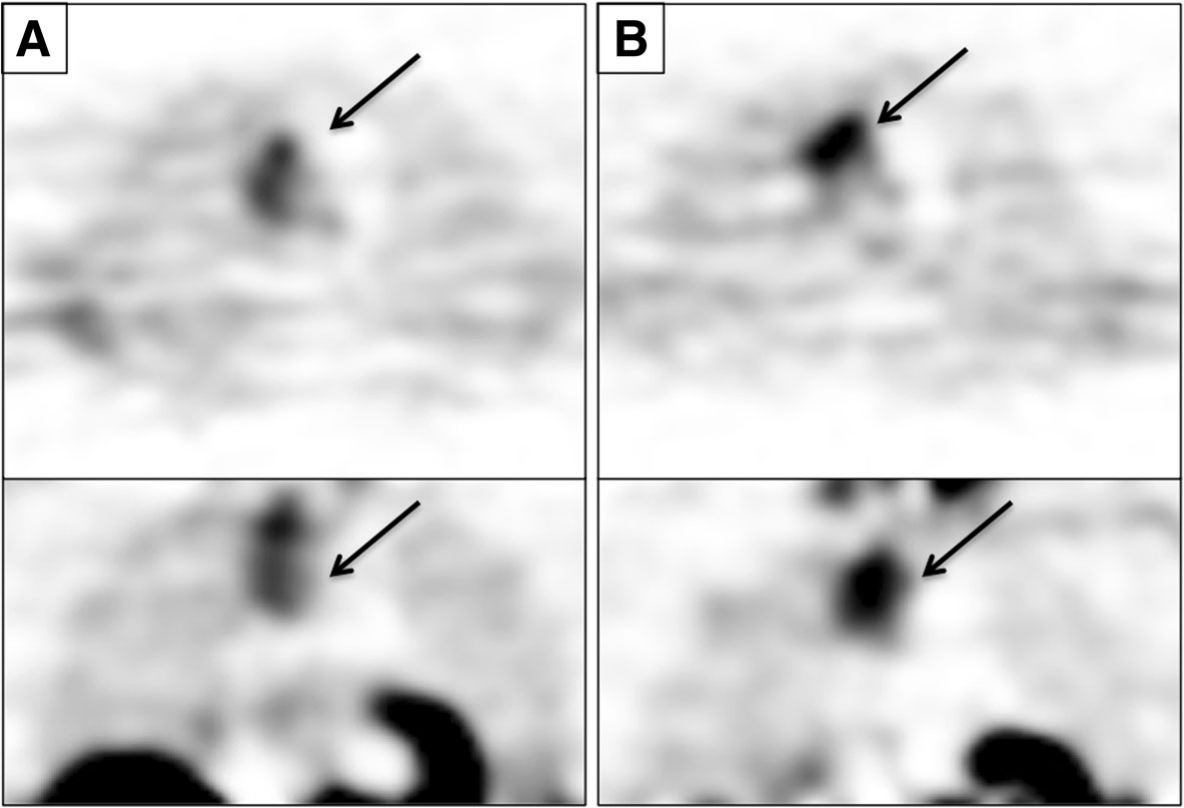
Tumor ^18^F-FMAU Uptake in Patient 7. Axial (top) and coronal (bottom) ^18^F-FMAU Images of a lung metastasis (arrow) in a patient with breast cancer at baseline (a) and after 1 day of capecitabine therapy (b). Tumor SUV_mean_ increased from 3.76 to 4.63
